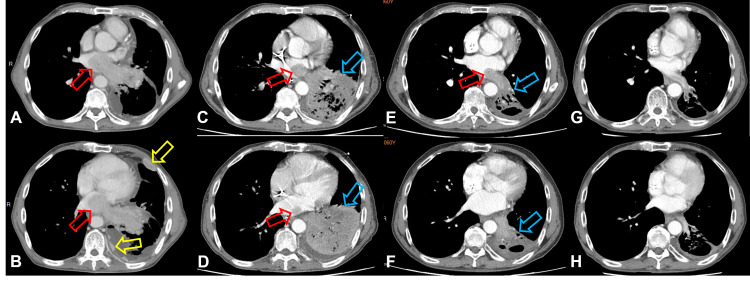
CT scan of chest during the whole treatment course. A and B. At initial presentation (May 11, 2020). C and D. Follow-up study after two courses of cisplatin/paclitaxel chemotherapy (Jul. 22, 2020). E and F. Follow-up study after four courses of cisplatin/paclitaxel chemotherapy (Oct. 7, 2020). G and H. Follow-up study after four additional courses of cisplatin/gemcitabine chemotherapy (Feb. 3, 2021). Red arrow: Tumor invasion into the left atrium from left inferior pulmonary veins. Yellow arrow: Pleural metastasis. Blue arrow: Left lower lobe metastatic tumor mixed with pneumonia consolidation.
